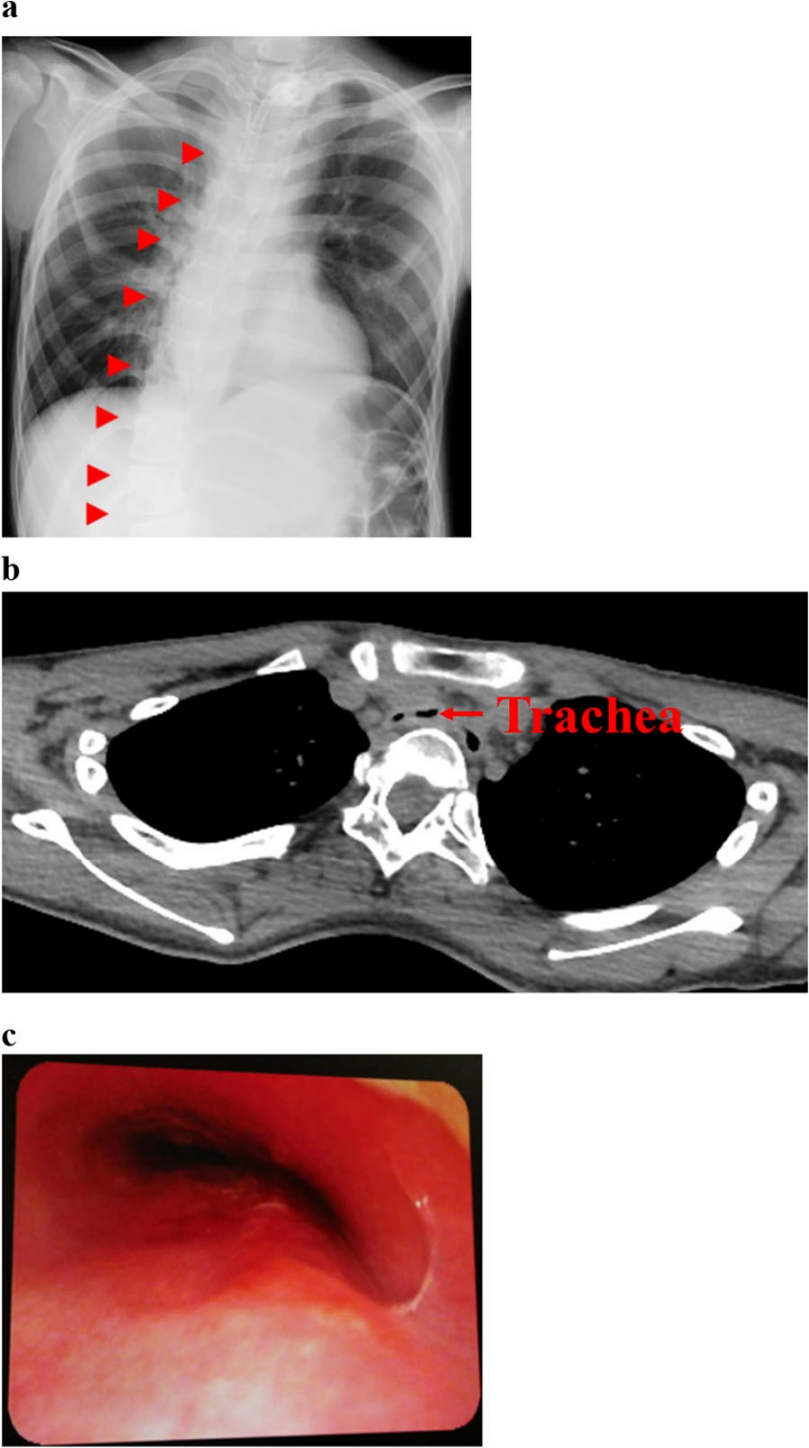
Preoperative findings. a Chest radiograph showing scoliosis (red arrowheads). b Computed tomography image demonstrates the stenosed trachea between the sternum, vertebral body, and innominate artery. c Bronchoscopy reveals a flattened trachea 4–5 cm caudal to the tracheostomy site, tracheal mucosa ulcers, and pulsation of the innominate artery on the anterior wall of the trachea
